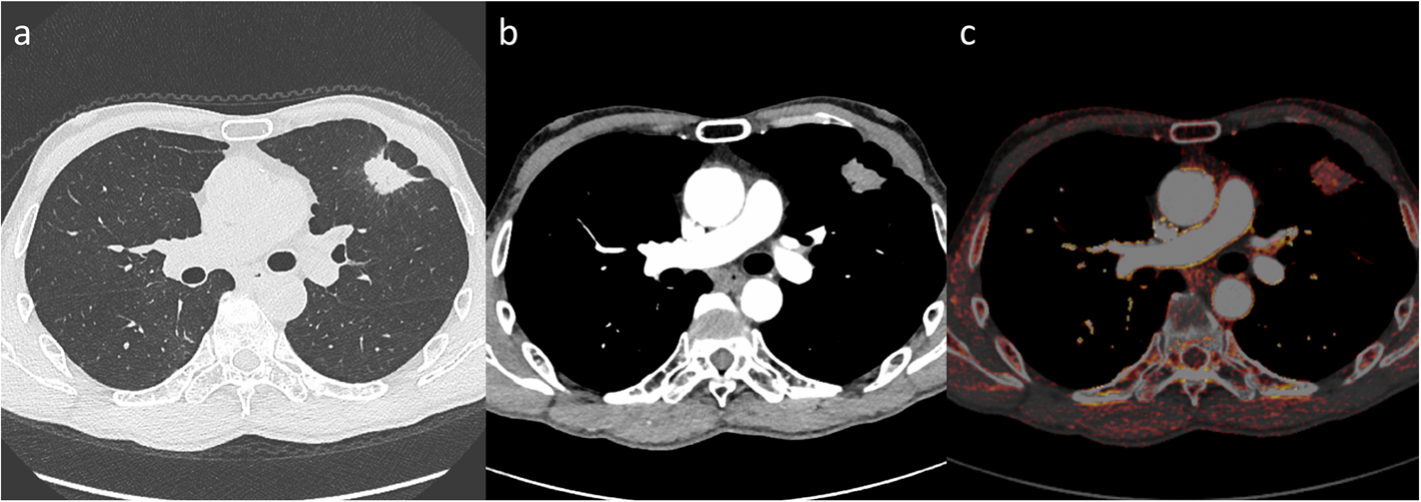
A 68-year-old male patient. An adenocarcinoma (pT2aN0) is observed in the left upper lobe. a Lung window setting. b Mediastinal window setting at the early phase of contrast-enhanced CT. c Iodine-enhanced image in contrast-enhanced CT. The 3D-IRA is 30 HU, and the corrected 3D-IRA is 4.59. The patient developed recurrence of adrenal metastases at 15 months after a left upper lobectomy
